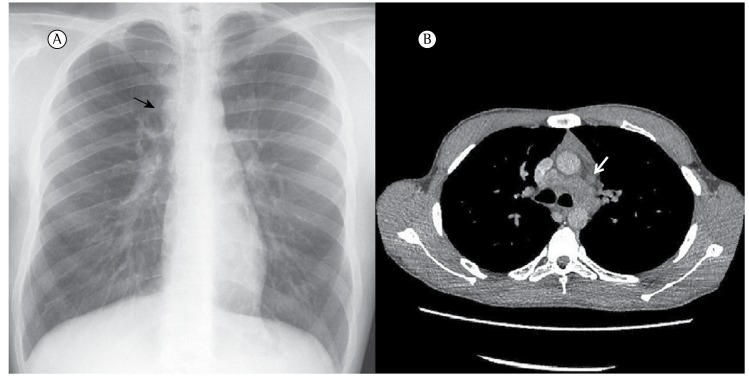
(A) Chest X-ray, posteroanterior view, showing a widened paratracheal stripe (arrow). (B) Highresolution CT of the chest revealing enlarged mediastinal lymph nodes (arrow).
